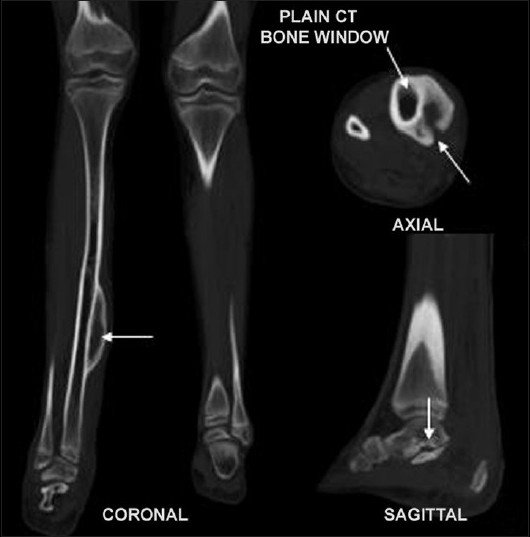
Plain computed tomography (bone window) demonstrates a large periosteal defect giving way to the infiltrating mass lesion, periosteal/ endosteal thickening (axial and coronal image-arrows) and cortical erosions at talocalcaneal joint (sagittal image-arrows)
